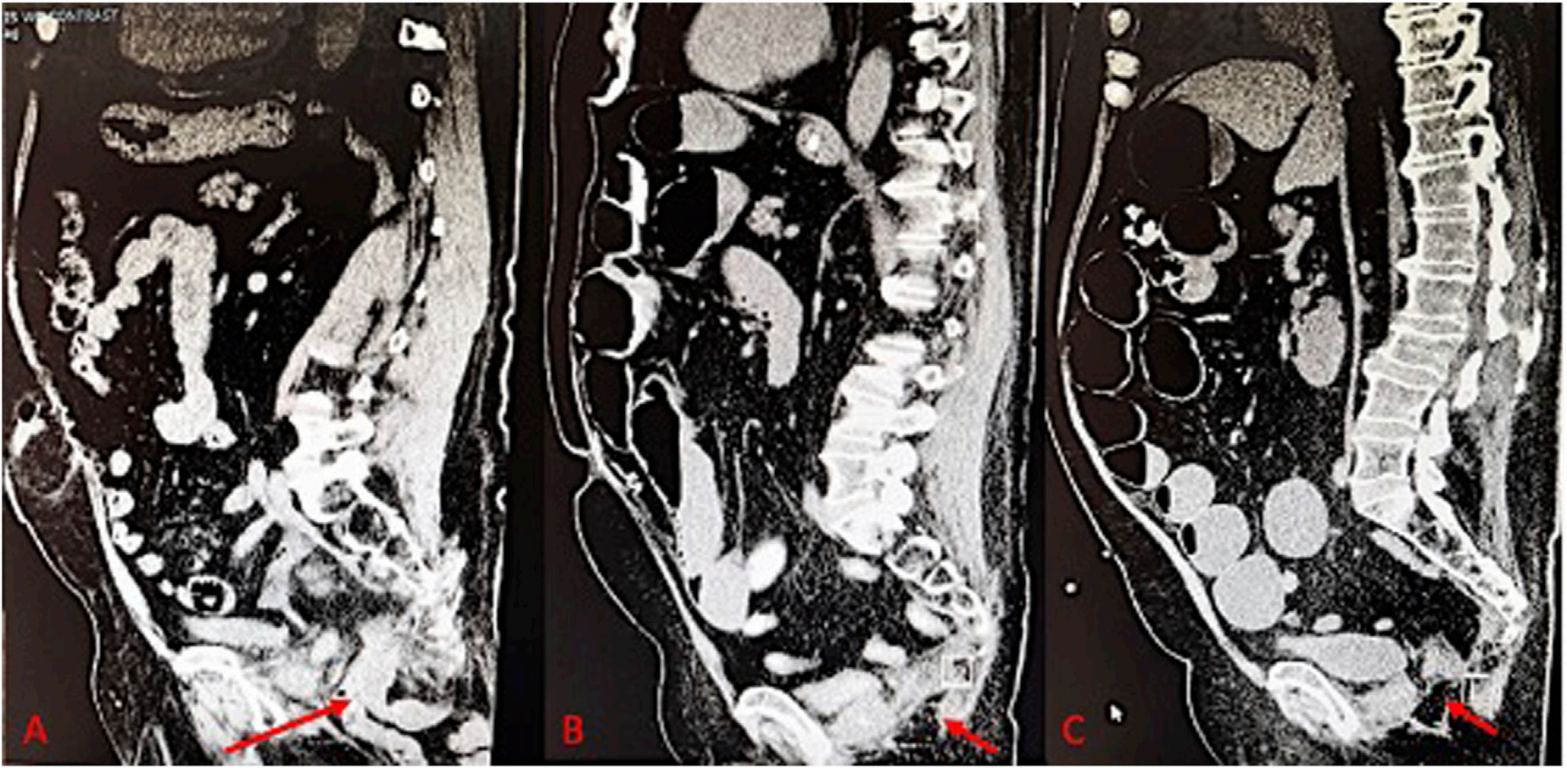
(A) Pre-operative CT scan of perineal hernia (red arrow); (B) One month post-operative CT image showing surgical correction of perineal hernia; (C) One year post-operative CT Image.
